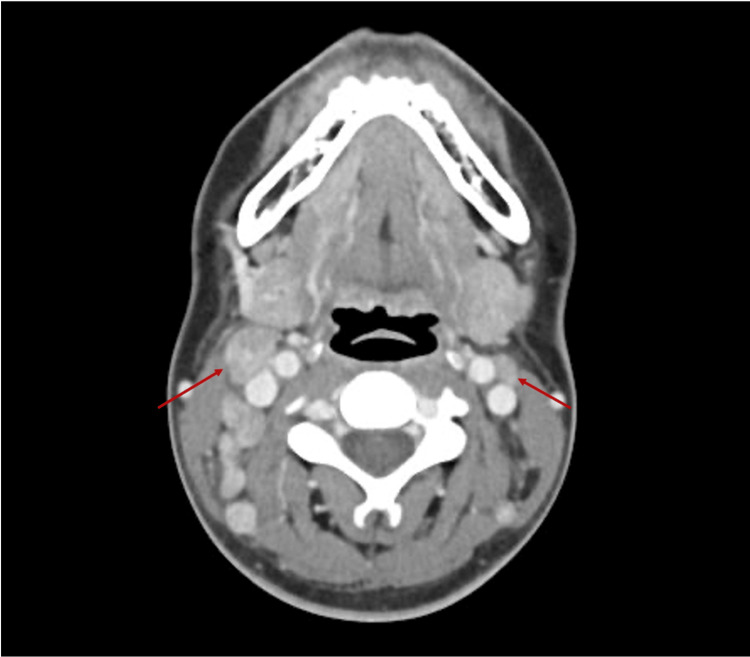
Second computed tomographic examination of the neck showing bilateral enhancing lymphadenopathy. Right cervical lymph nodes are more enlarged than left cervical lymph nodes (arrows).
